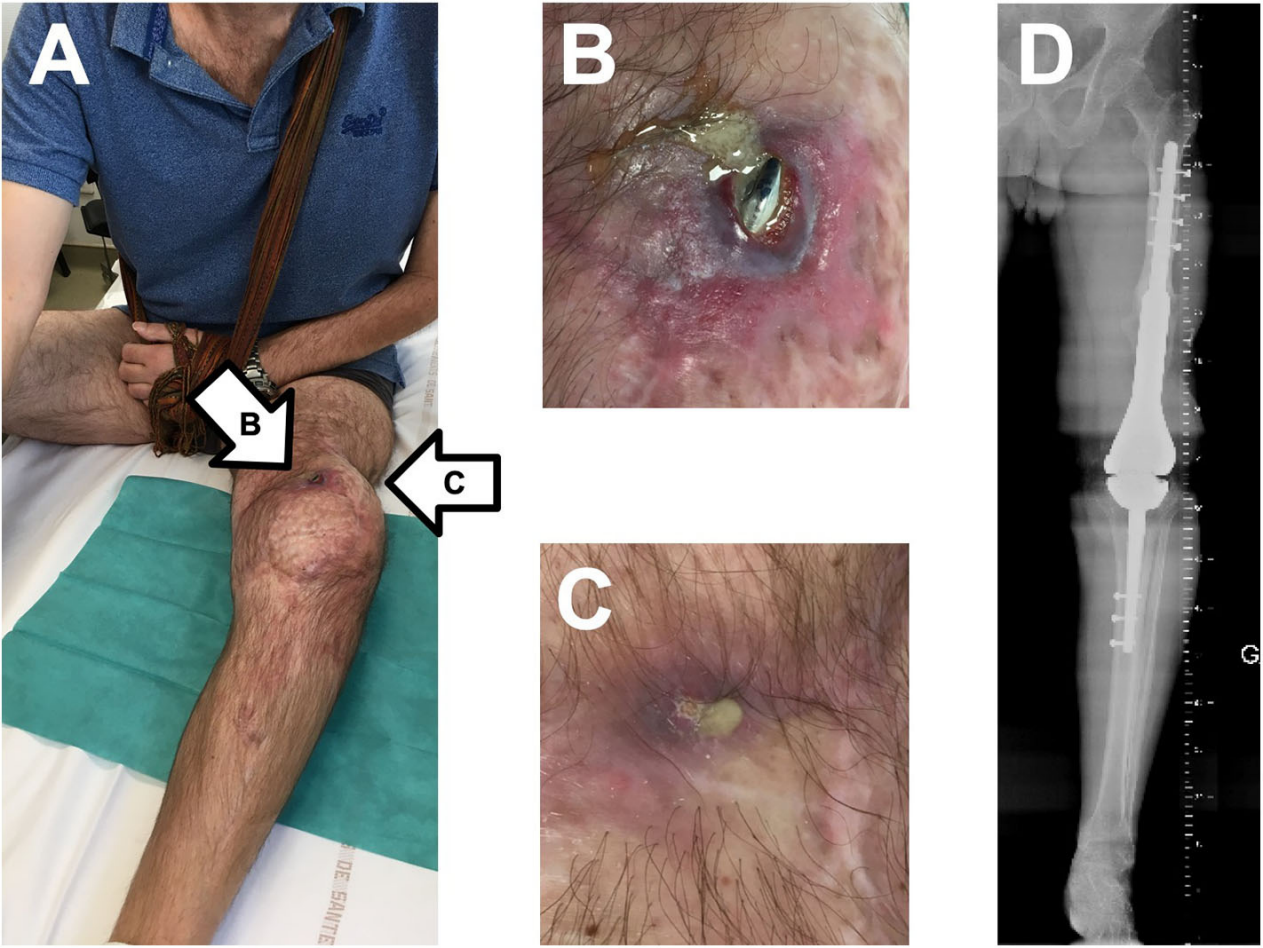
Clinical and X-ray status of the patient at baseline, with two fistulas regarding the femoral part of the megaprosthesis (A), with purulent discharge and prosthesis exposition (B,C), and without prosthesis loosening on X-ray (D).
